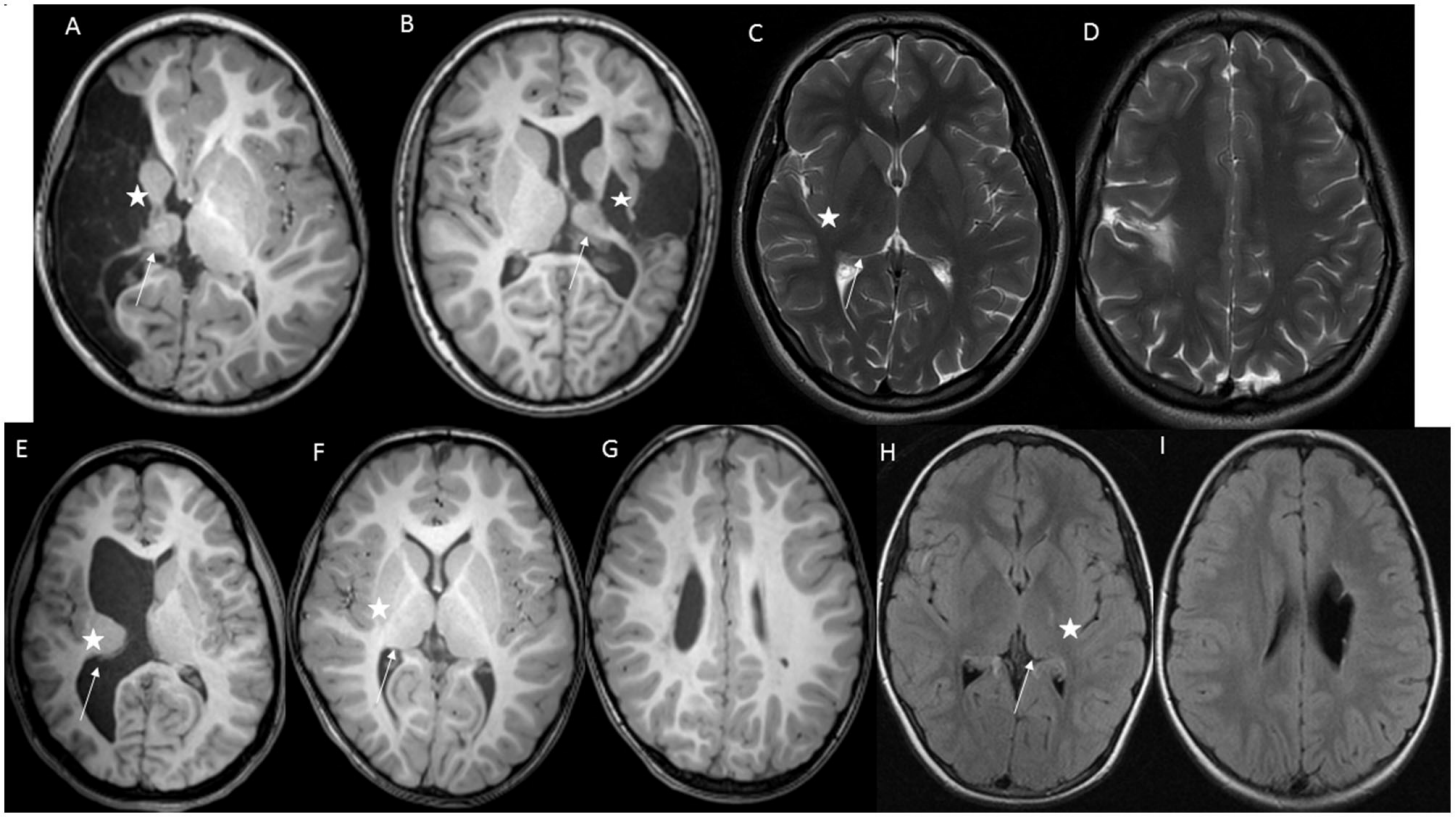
Individual MRI findings in children with perinatal stroke. Basal ganglia are marked with an asterisk, and the thalamus is marked with an arrow. (A) A girl at age 5 with neonatal AIS, right-side proximal middle cerebral artery stroke, and epilepsy. Axial T1-weighted MRI image shows the ipsilesional small size of the basal ganglia and thalamus. (B) A boy at age 14 with neonatal AIS and left-side proximal middle cerebral artery stroke, and IED. Axial T1-weighted MRI image shows the ipsilesional small size of the basal ganglia and thalamus. (C, D) A girl at age 16 with neonatal AIS and left-side anterior trunk of middle cerebral artery stroke without epilepsy. Axial PROPELLER T2-weighted MRI image shows the ipsilesional normal size of the basal ganglia and thalamus. (E) A girl at age 17 with large right-side presumed PVI and epilepsy. Axial T1-weighted MRI image shows right-side periventricular damage and ipsilesional small size of the basal ganglia and thalamus. (F, G) A girl at age 7 with right-side presumed PVI and IED. Axial T1-weighted MRI image shows right-side periventricular damage and ipsilesional small size of the thalamus and normal basal ganglia. (H, I) A girl at age 11 with left-side presumed PVI and without epilepsy. Axial FLAIR MRI image shows left-side periventricular damage and ipsilesional minimally smaller size of the thalamus and normal basal ganglia.
